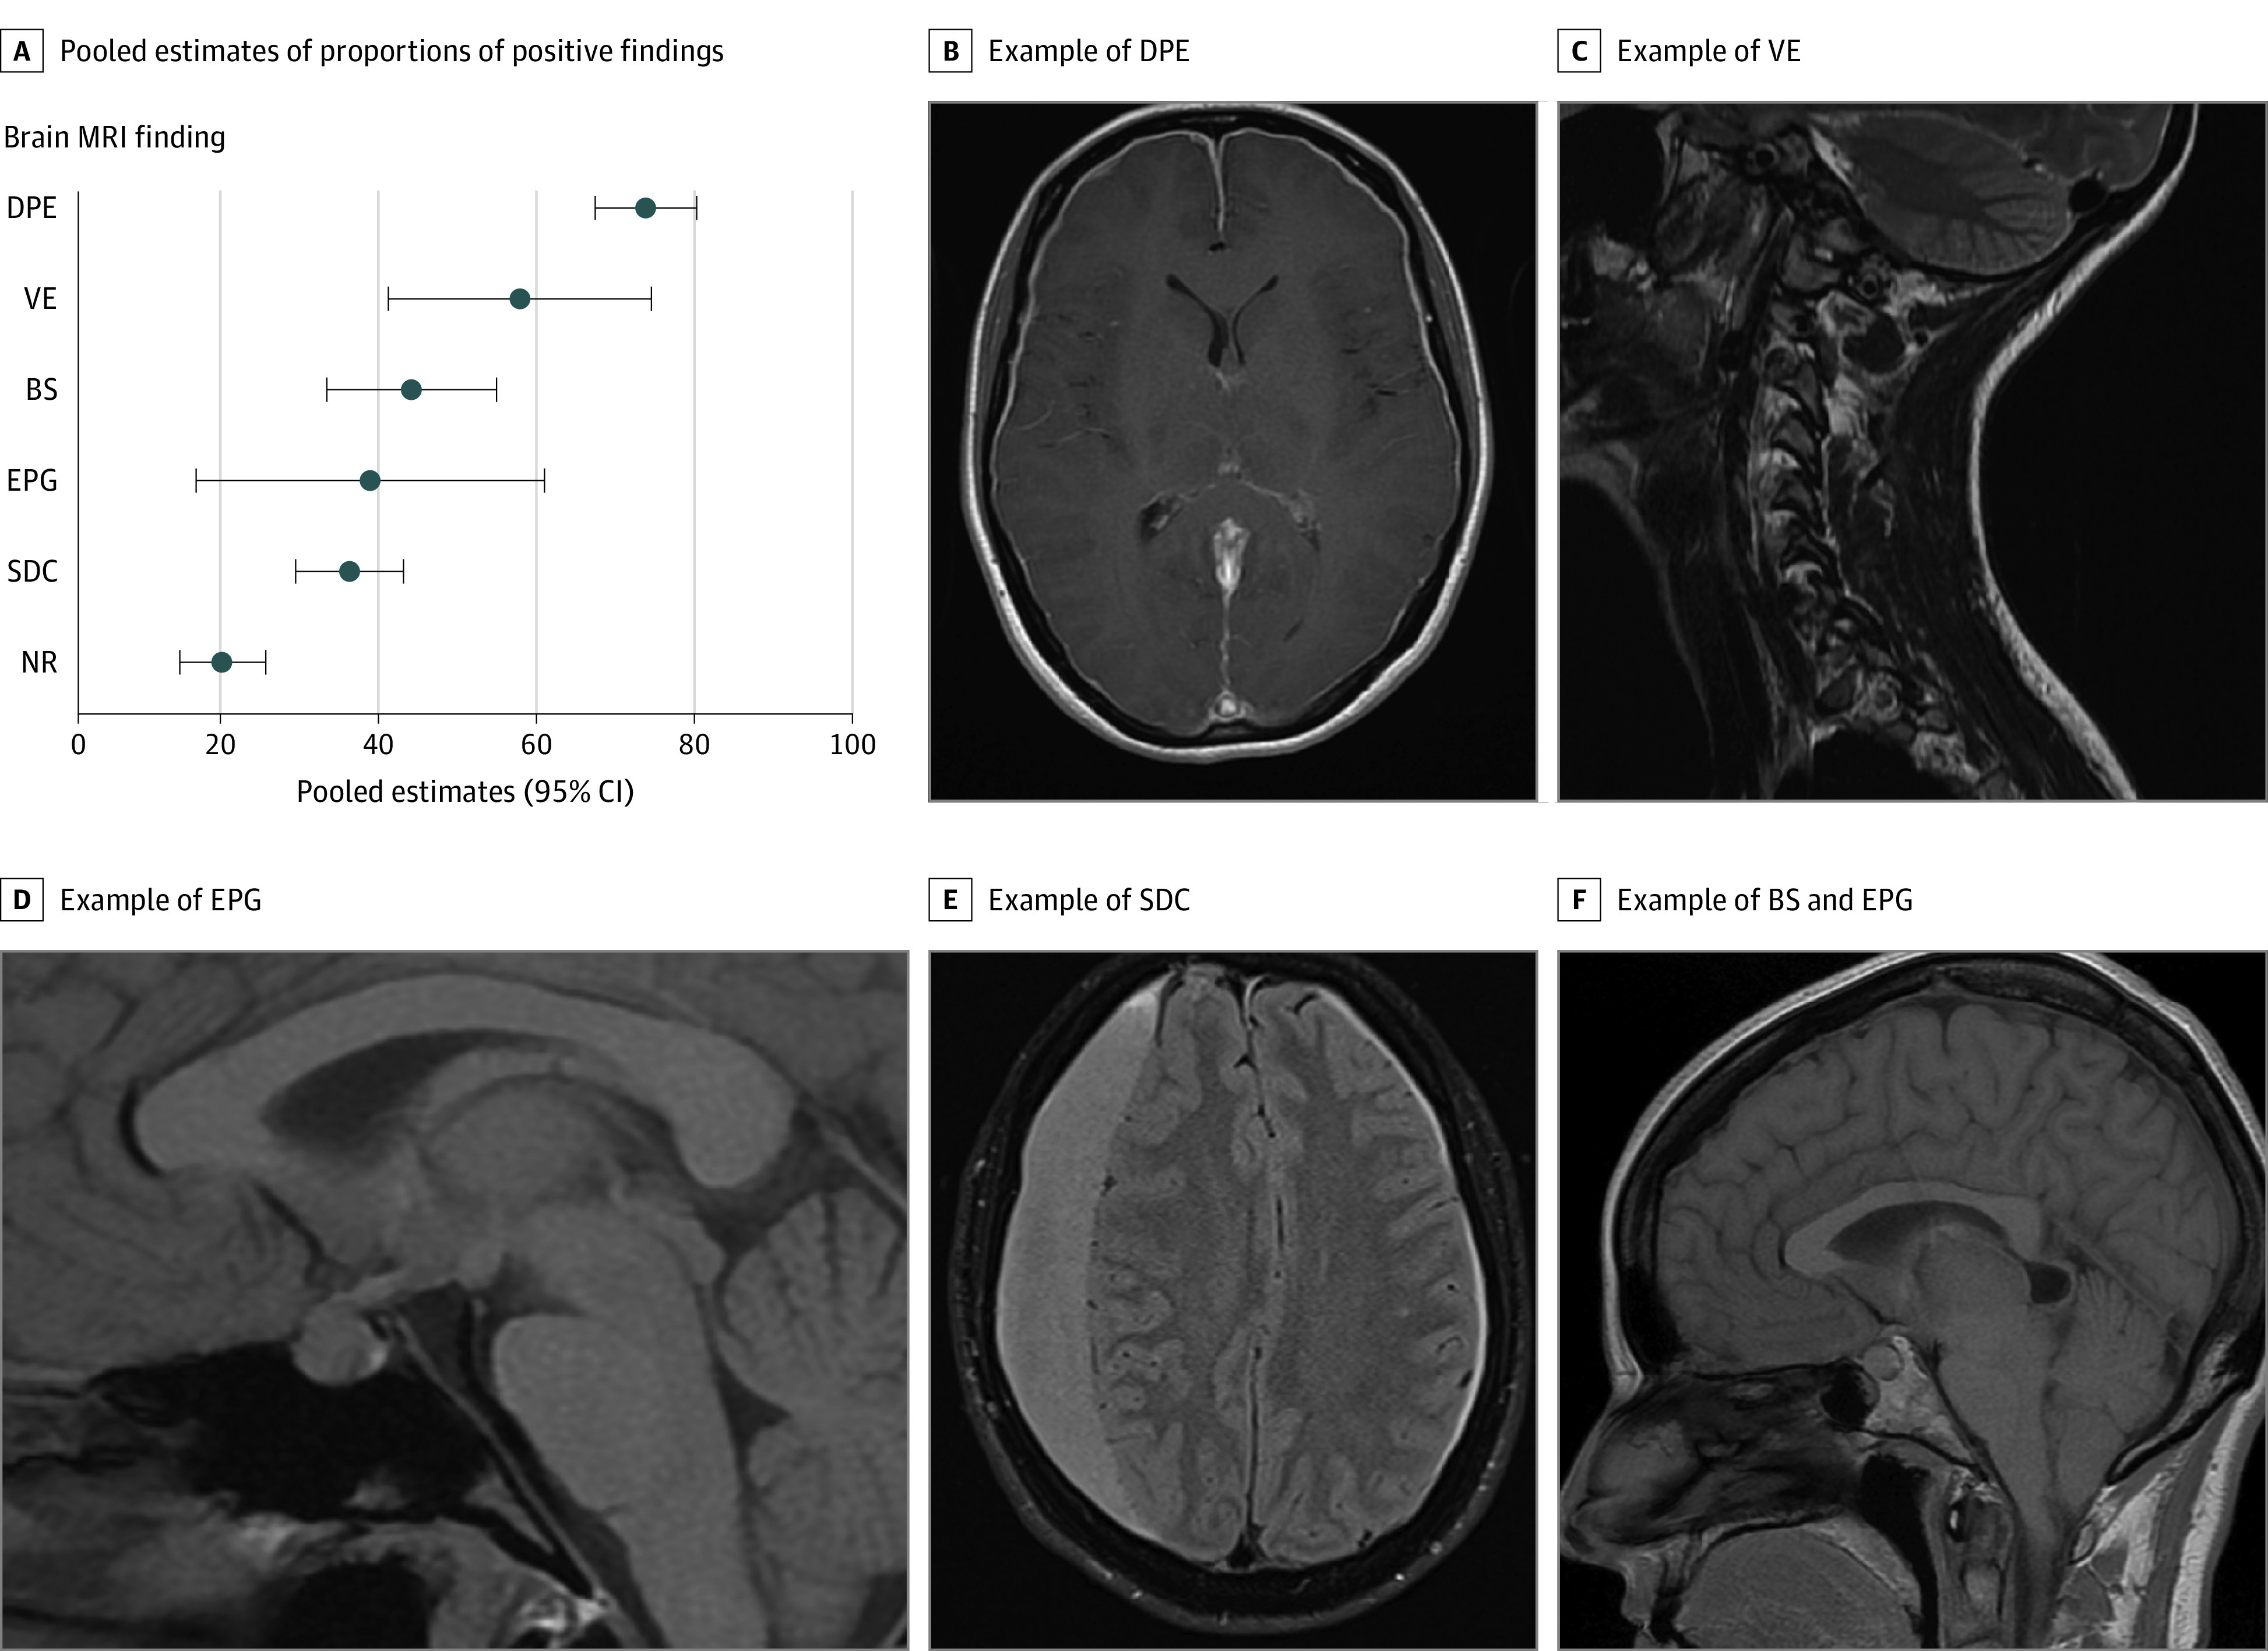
Brain Magnetic Resonance Imaging (MRI) Findings of Spontaneous Intracranial Hypotension A, Pooled estimates of proportions of positive findings in spontaneous intracranial hypotension. B, Example of diffuse pachymeningeal enhancement (DPE) in T1-weighted axial MRI sequence. C, Example of venous engorgement (VE, transverse sinus venous distension sign) in T2-weighted sagittal MRI sequence. D, Example of enlarged pituitary gland (EPG) in T1-weighted sagittal MRI sequence. E, Example of subdural collection (SDC) in T2-weighted axial MRI sequence. F, Example of brain sagging (BS) and enlarged pituitary gland (EPG) in T1-weighted sagittal MRI sequence. NR indicates normal.
